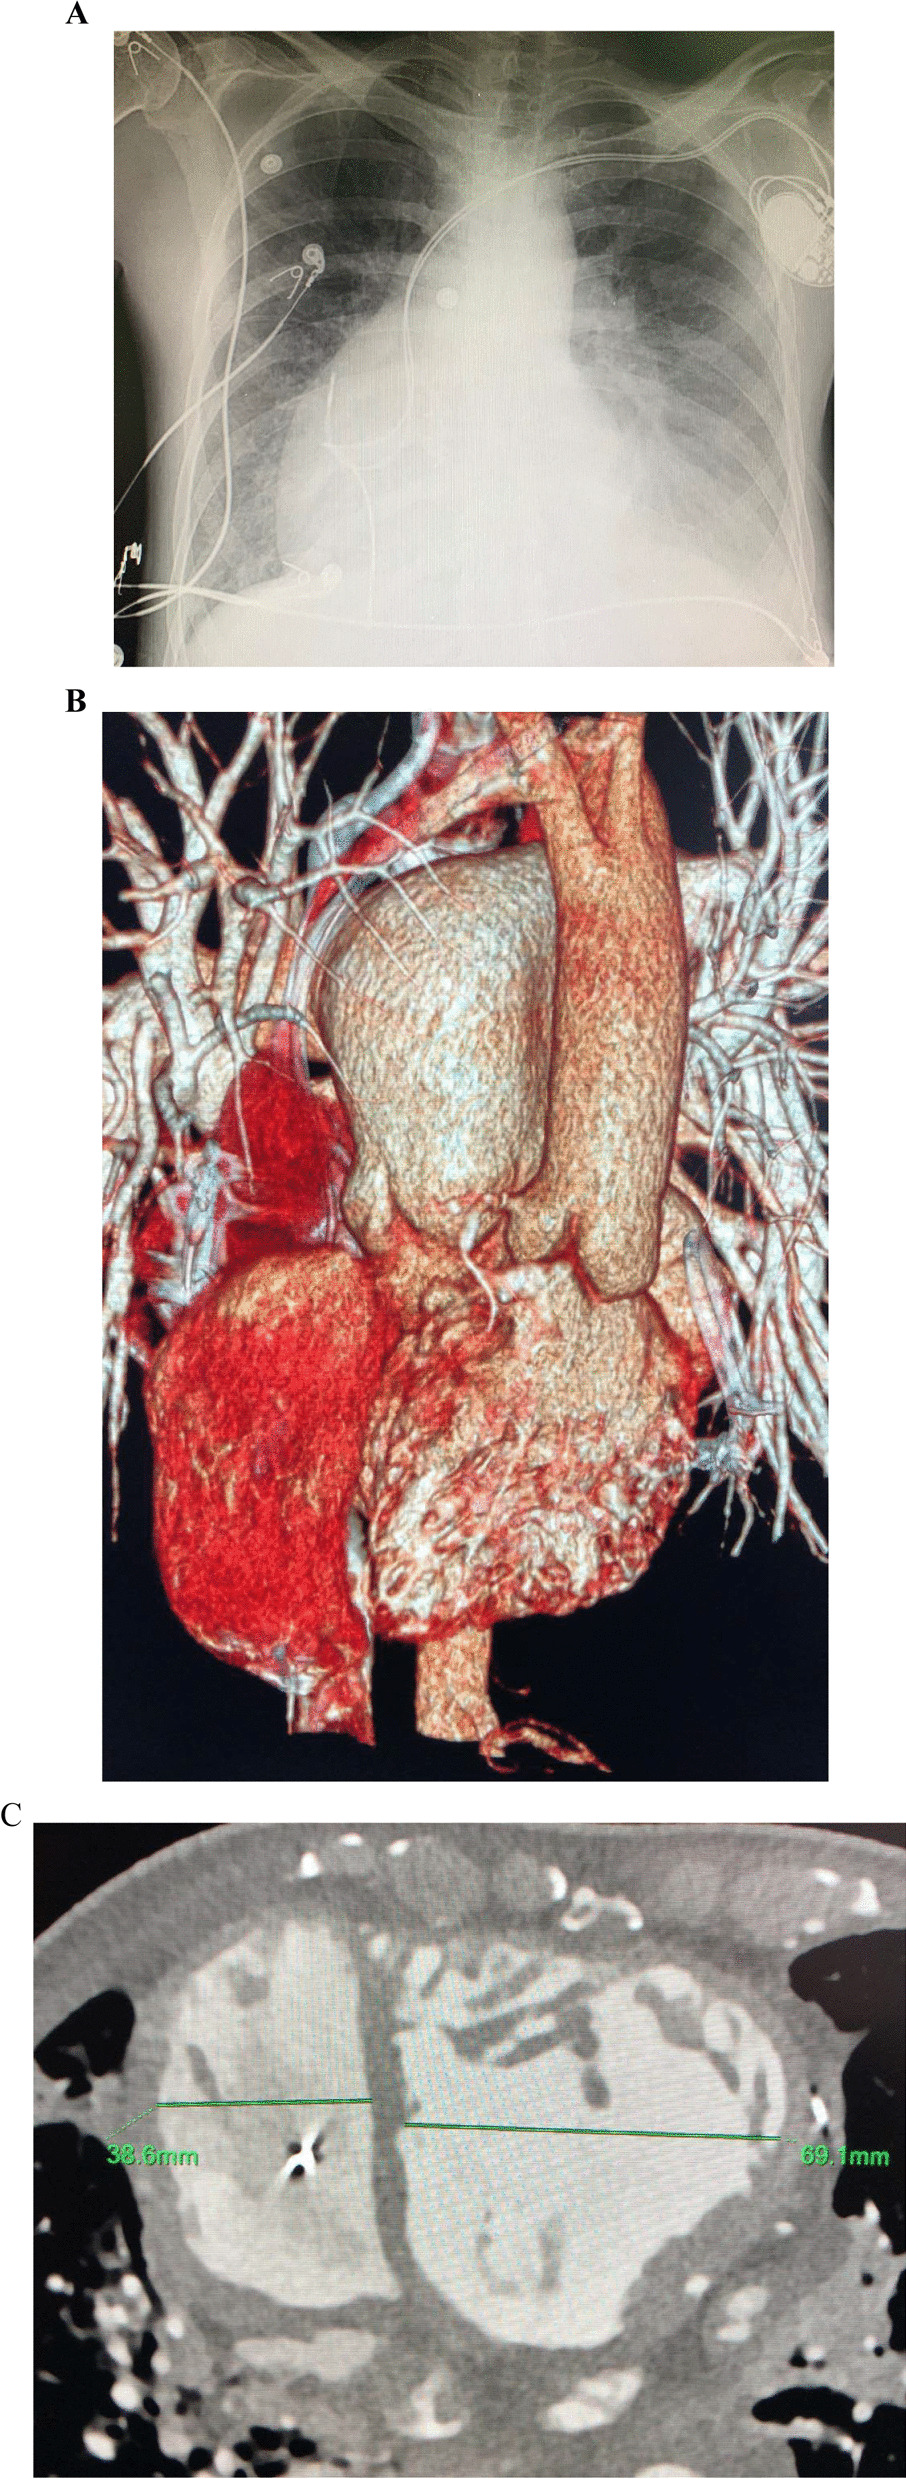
A Chest X-Ray of a ccTGA patient with no dextrocardia, the LV can be seen on the right side of the chest. B 3D cardiac CT reconstruction of the heart and the great vessels; On the left side a distended PA and on the right side a “thin” aorta. C The dimensions of both ventricles are depicted the systemic RV and it’s trabeculation are seen on the left side of the image
